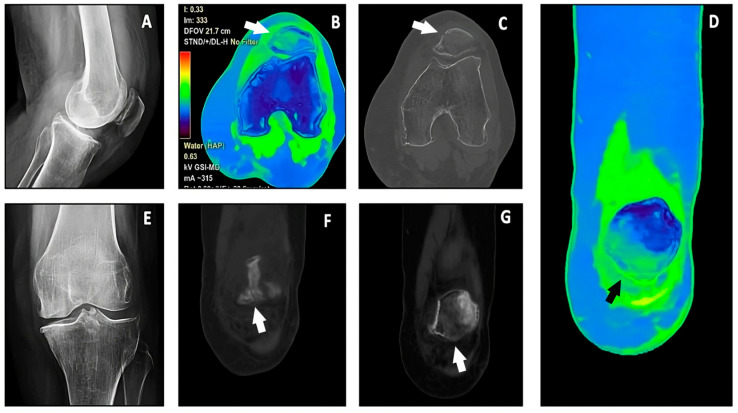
A patient referred for direct trauma to the left lateral condyle-patella, with severe pain on acupressure on the lateral condyle and functional impotence. A plain radiograph of the left knee showing a suspected compound fracture of the lower edge of the left patella (white arrows) (A,E), further confirmed by the bone reconstruction of axial (C) and coronal CT images (F,G). VNHAP axial and coronal color-coded maps confirmed the presence of bone marrow edema at the fracture site (B,D).
